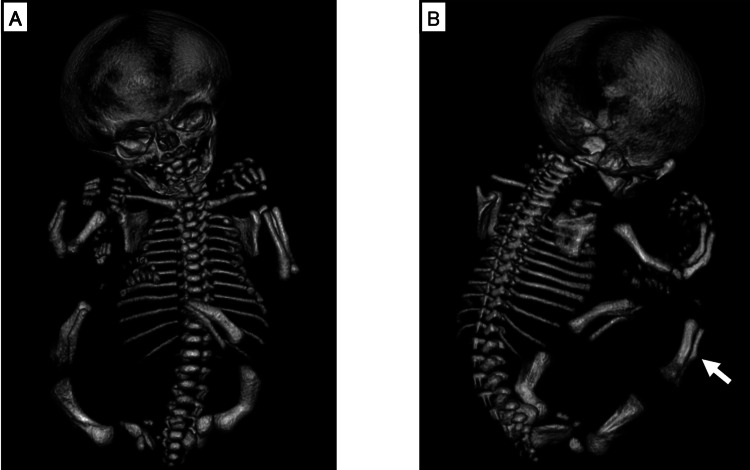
Fetal 3D-CT at 32 weeks gestation A: Deformities of long bones (bowed radius, ulna, femur, and tibia). B: The bone spur of the fibula (arrow). 3D-CT: three-dimensional computed tomography
